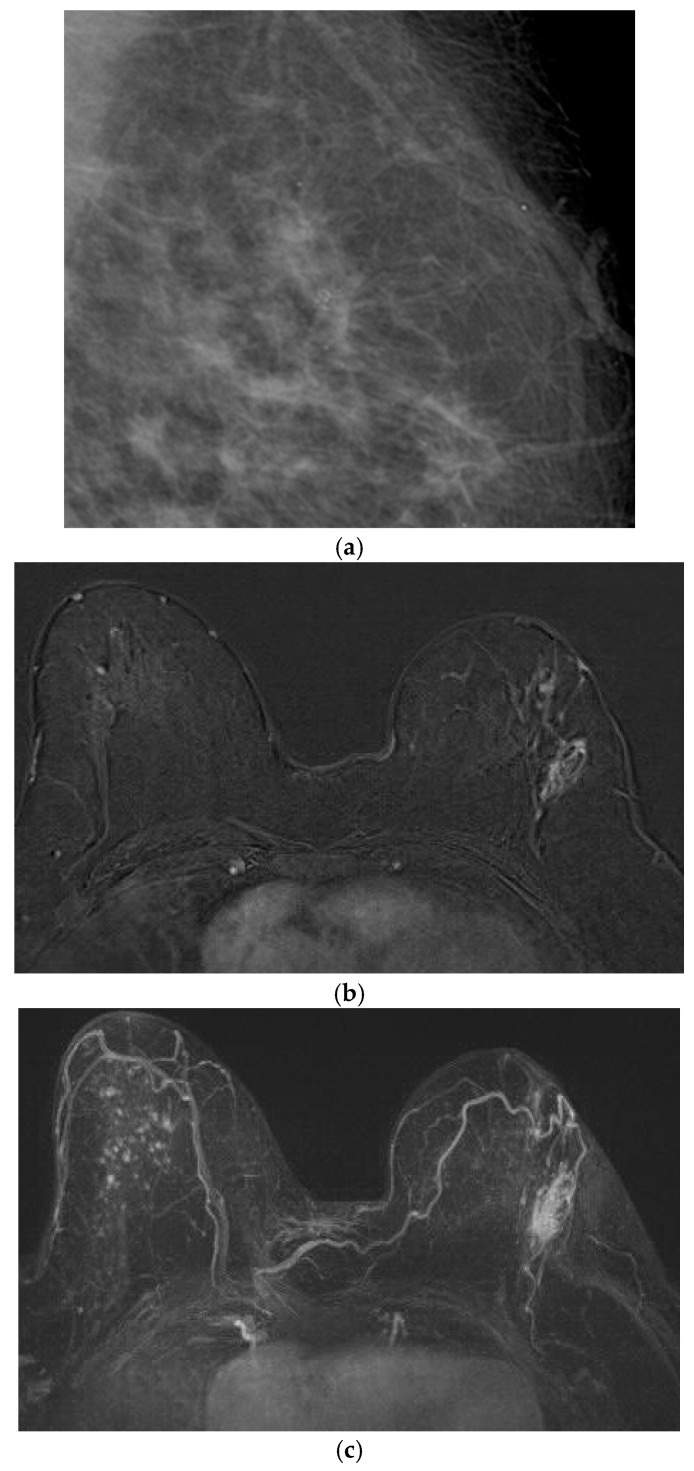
A 64-year-old woman previously treated with breast-conserving surgery and radiotherapy (6 years earlier) shows a new area of parenchymal consolidation with associated pleomorphic calcifications (a). Contrast-enhanced T1-weight MRI and MIP reconstructions demonstrate a 2 cm non-mass enhancement located at the site of the surgical scar, findings compatible with disease recurrence (b,c).
